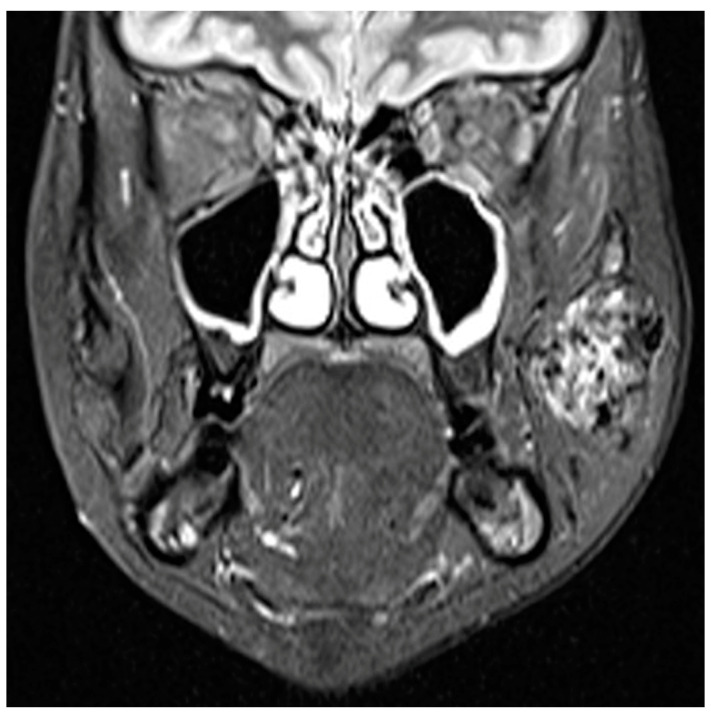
MRI imaging of a vascular tumor of the left side of the face with T2 hypersignal suggesting an infantile hemangioma.
